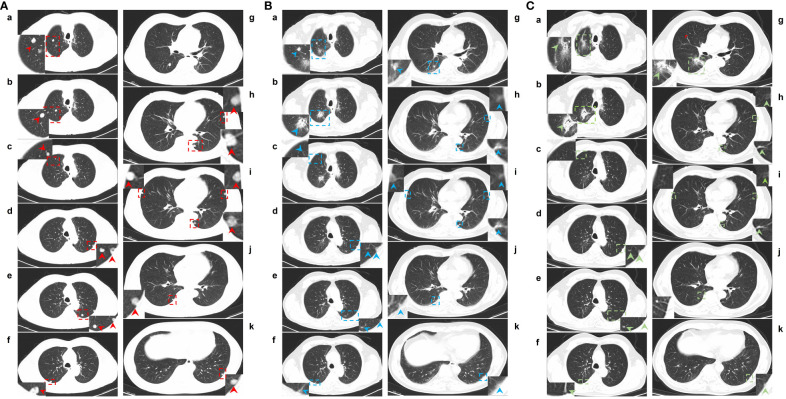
CT images of lung metastases at baseline and after two cycles and 18 cycles of camrelizumab plus SBRT. (A), a-k: Lung window of CT images for lung metastases at baseline. (B), a-k: Lung windows of CT images of lung metastases after 2 cycles of camrelizumab plus SBRT. (C), a-k: Lung windows of CT images for lung metastases after 18 cycles of camrelizumab plus SBRT. In total, 13 metastatic nodules were located in the lungs. One nodule located in the right upper lung (Ab) and one in the right lower lung (Ag) received SBRT at 45 Gy/5 f, one day for each alternate, twelve days after the first cycle of camrelizumab. The second cycle of camrelizumab was administered 2 weeks after SBRT, and repeat thoracic CT was delivered 4 weeks later. On the repeat CT scan, the metastases that received SBRT showed fibrosis, fuzziness, and consolidation changes (Bb and Bg). For most metastases that did not receive SBRT, an obvious volume decrease and fuzzy change were observed, including one nodule that completely vanished (Bc). Other 16 cycles of camrelizumab were administered; the repeat CT showed more obvious fibrosis, fuzziness, and consolidation changes in the target nodules, and the nontarget nodule received radiation at a relatively high dose (Ca and Cf). The volume of other nontarget nodules continued to decrease, and the fuzzy change was more obvious, including another nodule that completely vanished (Cj). Red, blue, and green arrows: metastases located in the lungs at baseline and after treatment. Red and blue dotted boxes: particular areas surrounding metastases, which are magnified and displayed on the side.
